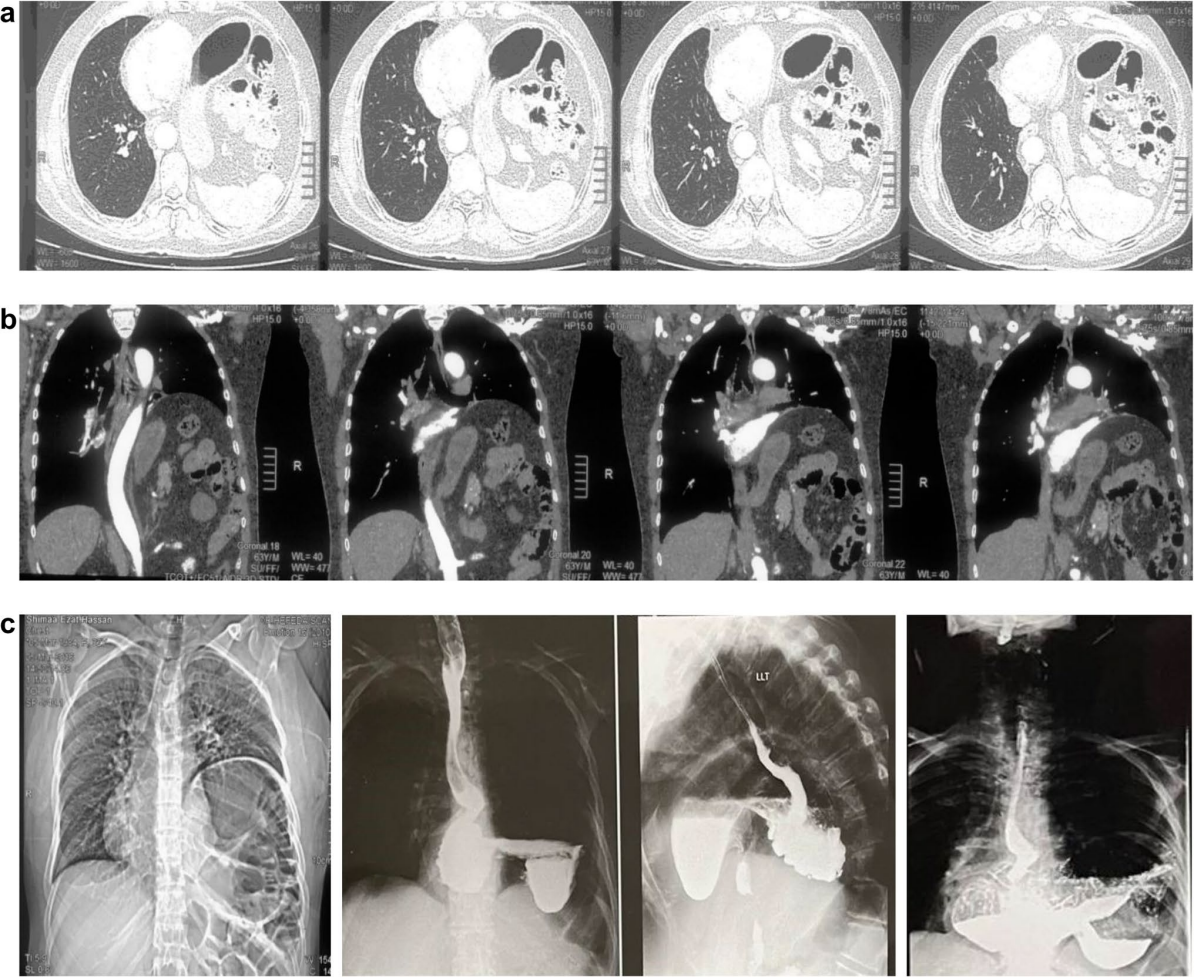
A, B CT evaluation of left diaphragmatic eventration. C, Chest X ray and barium study with finding of organaxial volvulus of the stomach
